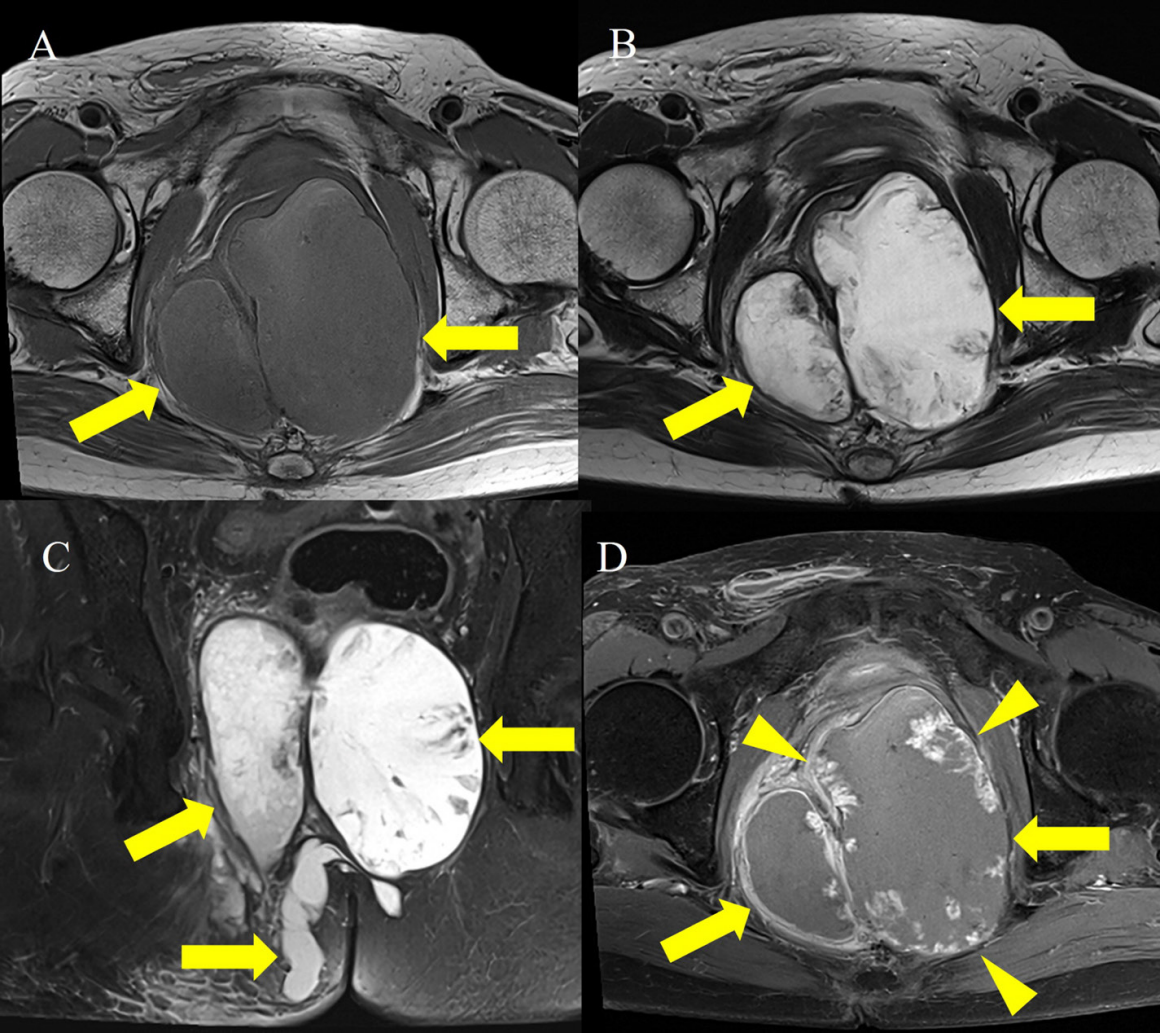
MRI images showing multilocular cystic mass with T1 hyperintensity and peripheral irregular enhancement.
